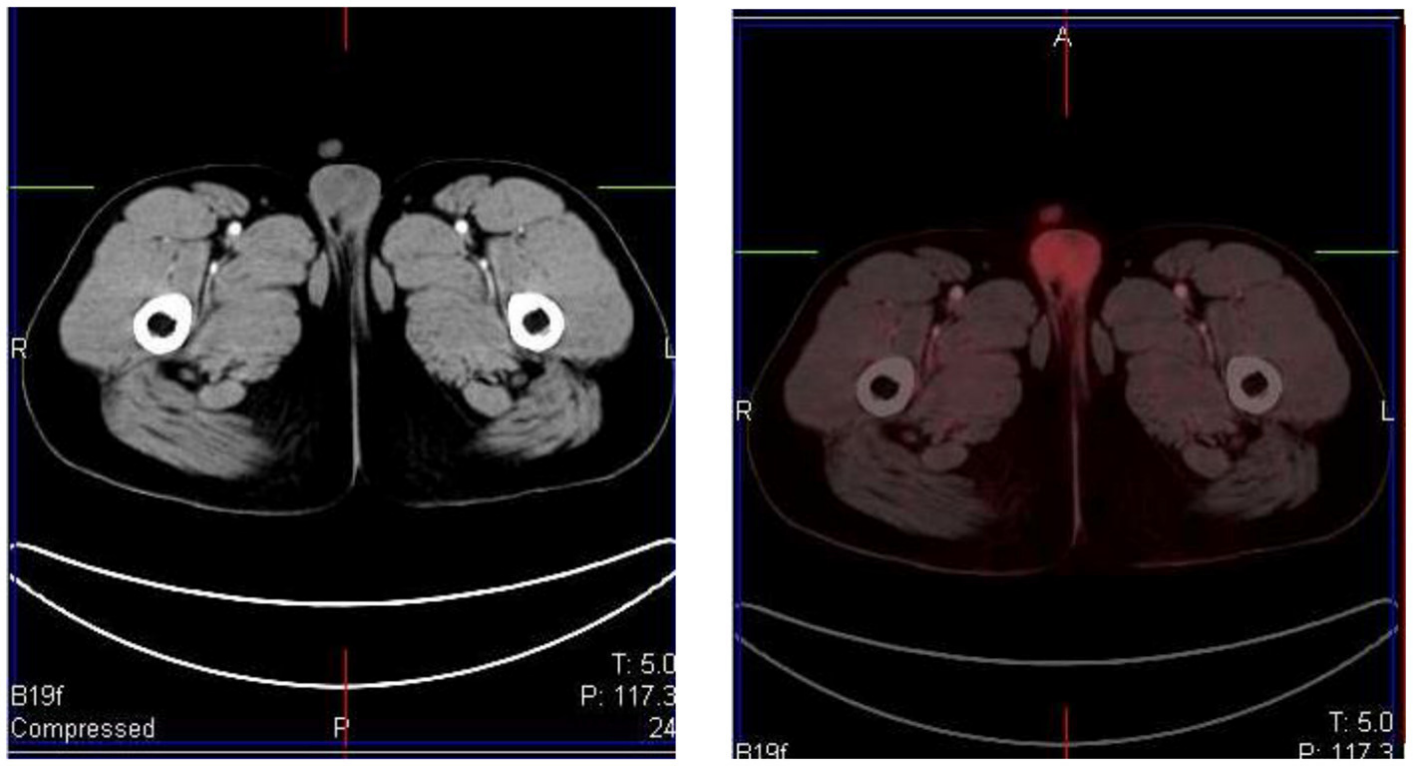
A 16 year old male with Hodgkin's lymphoma referred to evaluate the end of treatment response to chemotherapy. Pubertal boys may have diffuse, increased and symmetrical uptake in the testes. No abnormality was seen at CT.
